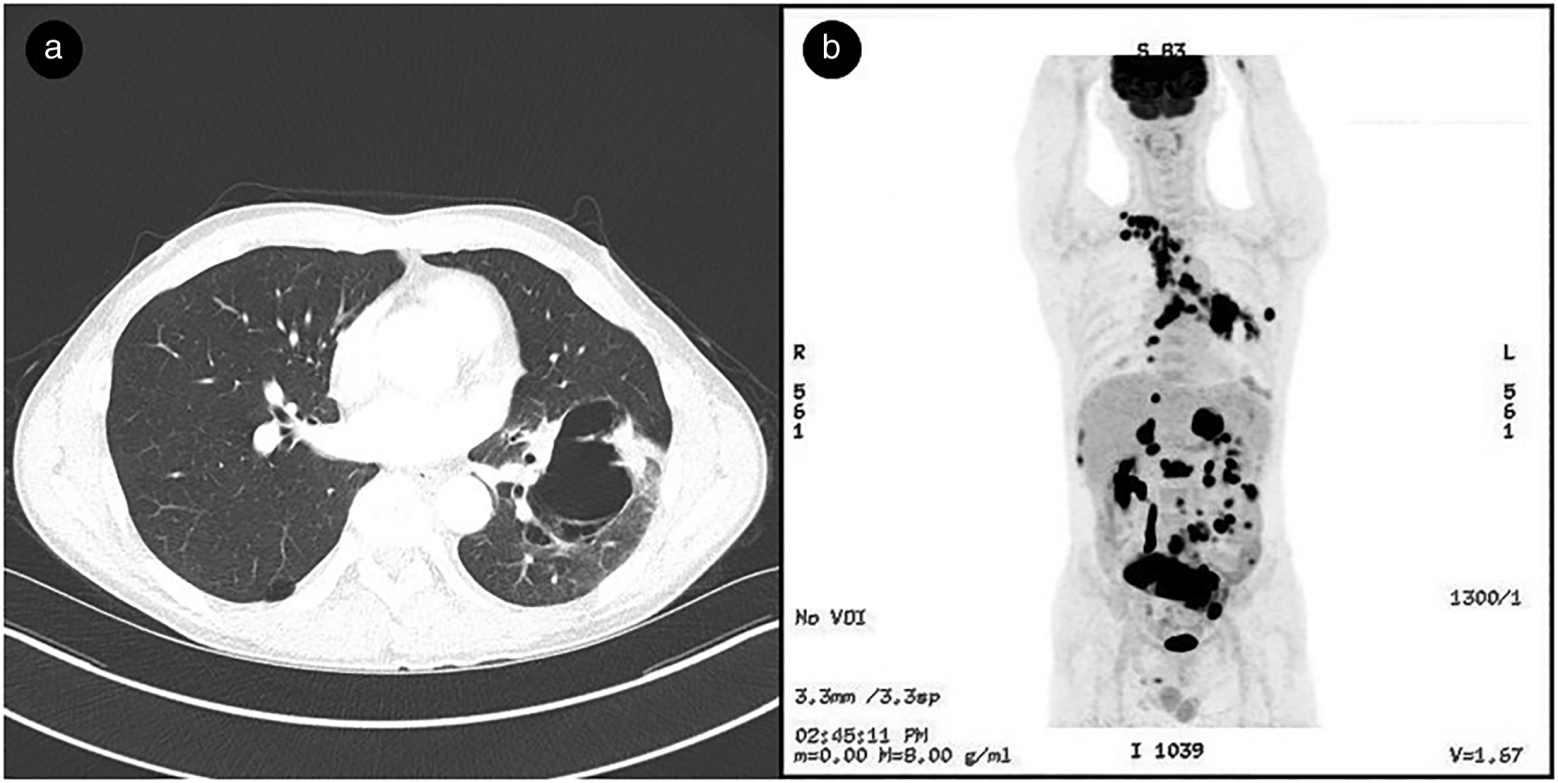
(a) Chest computed tomography revealed a cavitary mass 8.7 cm in size in the superior segment of the left lower lobe and multiple mediastinal lymphadenopathy. (b) Positron emission tomography‐computed tomography (PET‐CT) revealed a typical pattern of metastasis for non‐small cell lung cancer (NSCLC).
